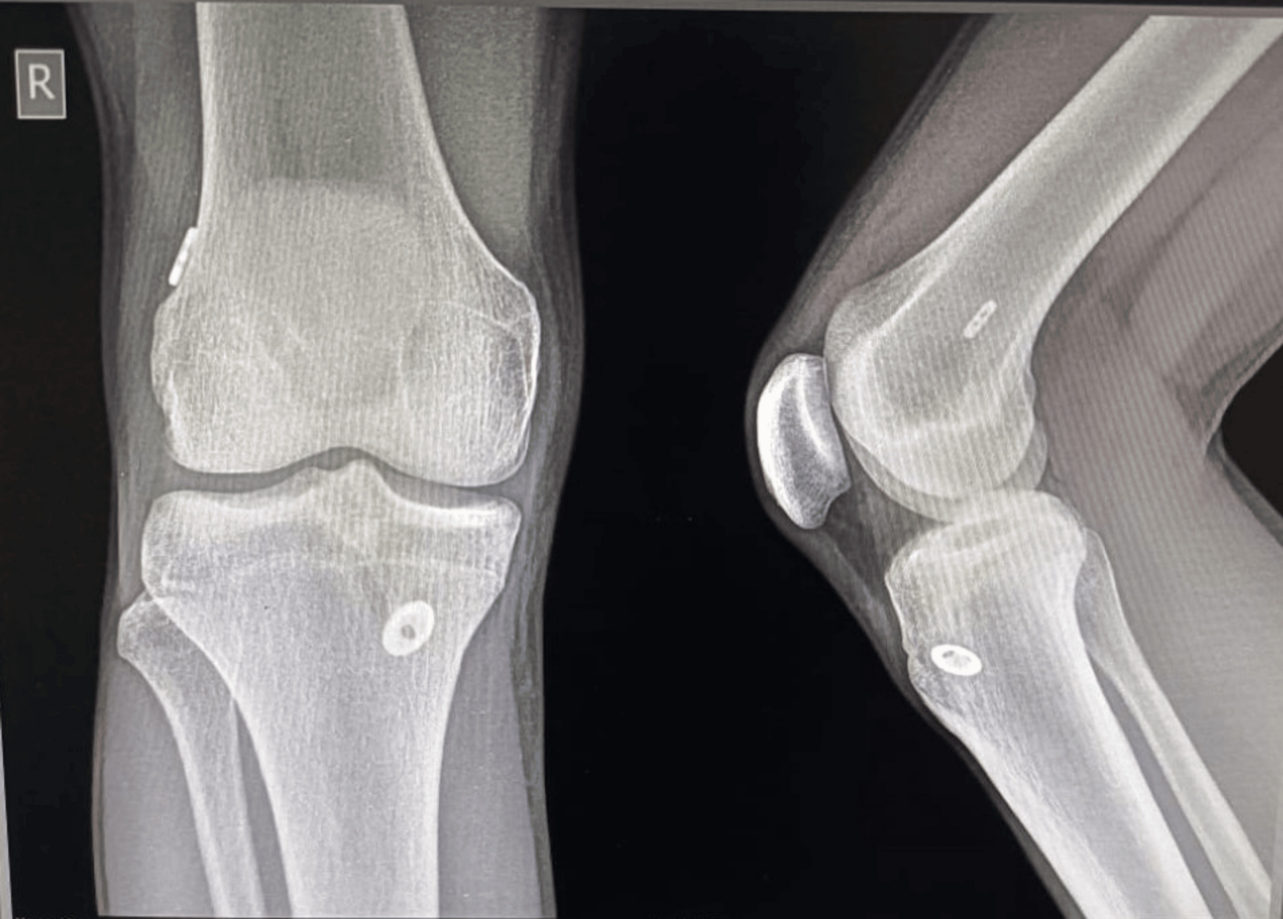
Knee radiograph of the same patient after 5 years
